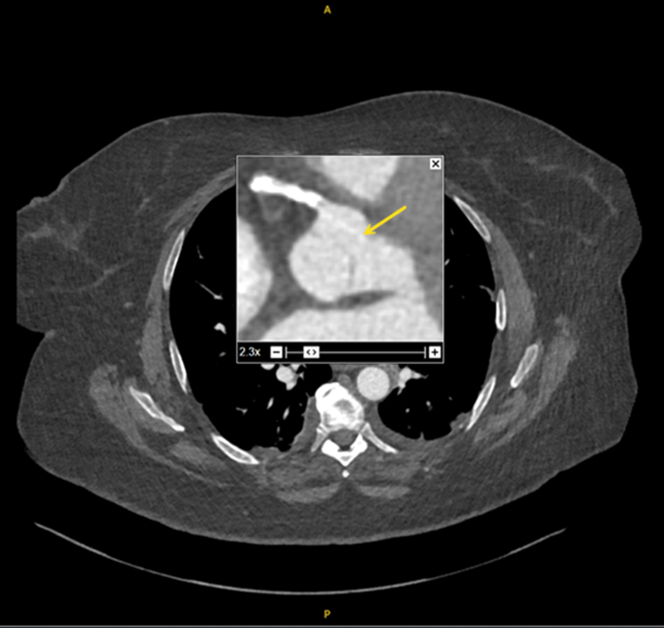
Computed Tomography Aorta Showing Subtle Hyperattenuating Linear Artefact Arising in the Right Coronary Artery Progressing Across the Right Coronary Cusp Suggesting a Protruding Wire
